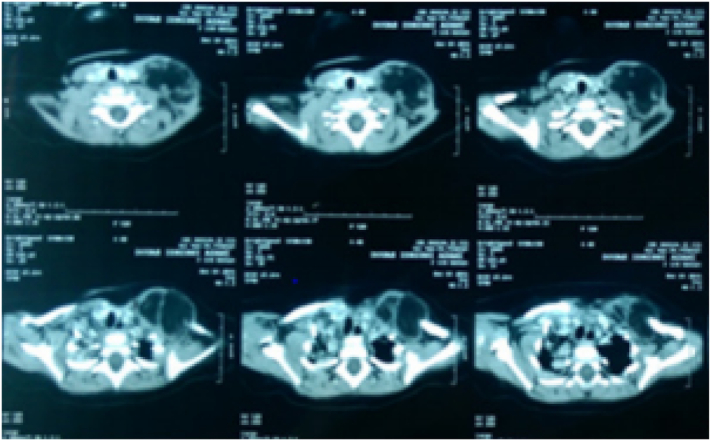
Axial neck CT scan section showing a well limited supraclavicular lobulated mass (62 × 43 × 41 mm) encroaching upon the sternocleidomastoid muscle.
